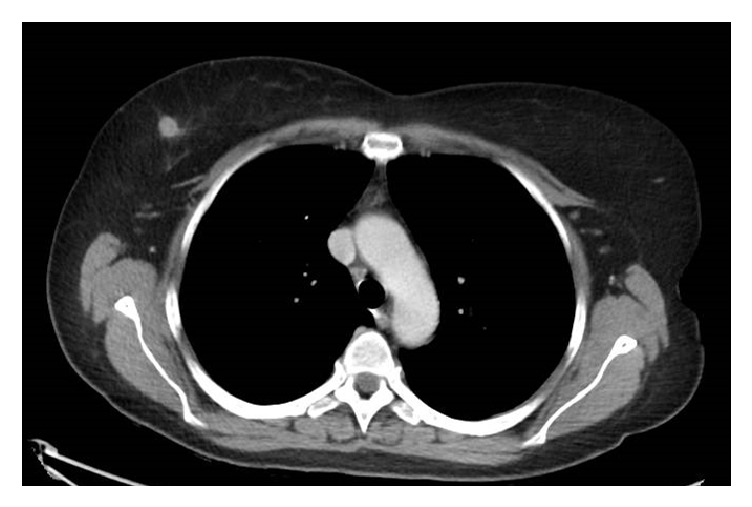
CT scan of the chest at the time of diagnosis demonstrated a round, hyperintense, 1.0 cm lesion in the upper outer quadrant of the right breast.
